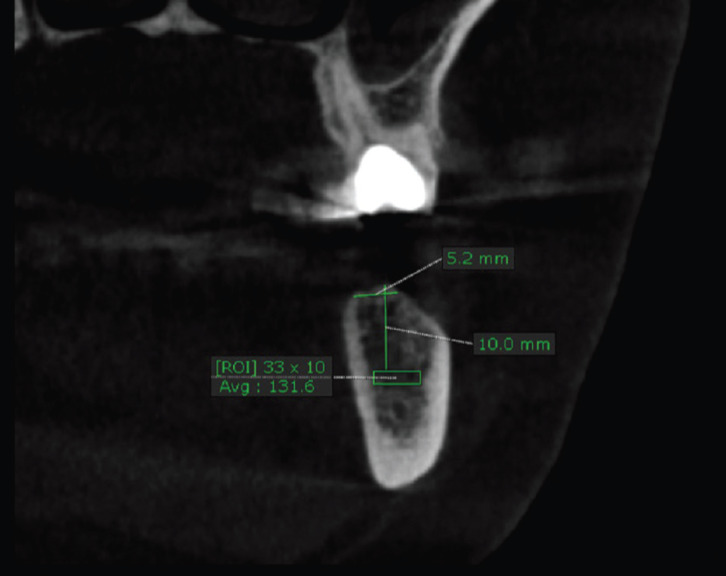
Preoperative coronal view of CBCT showing the average bone density of the area apical to the proposed implant length (131.6 Hounsfield unit).
